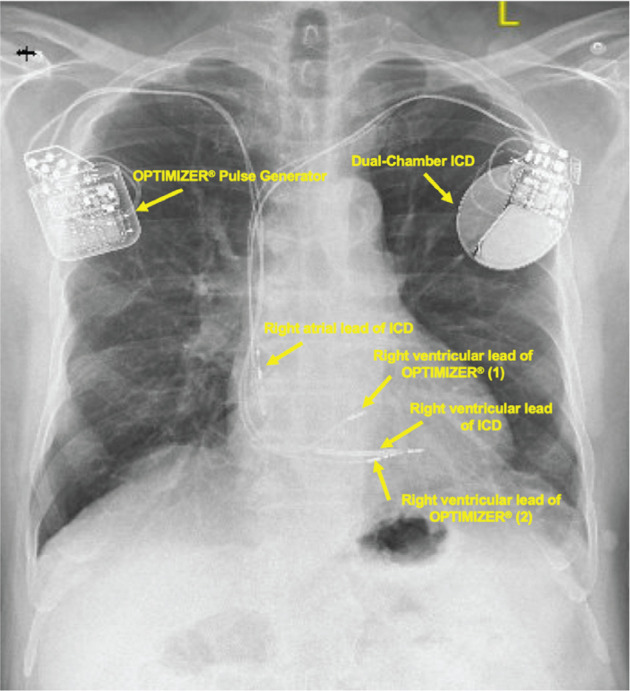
Chest X-ray of the patient with the two-lead OPTIMIZER^®^ Smart Implantable Pulse Generator visible in the upper right pectoral region and a dual-chamber implantable cardioverter-defibrillator visible in the left upper pectoral region. *Abbreviation:* ICD, implantable cardioverter-defibrillator.
